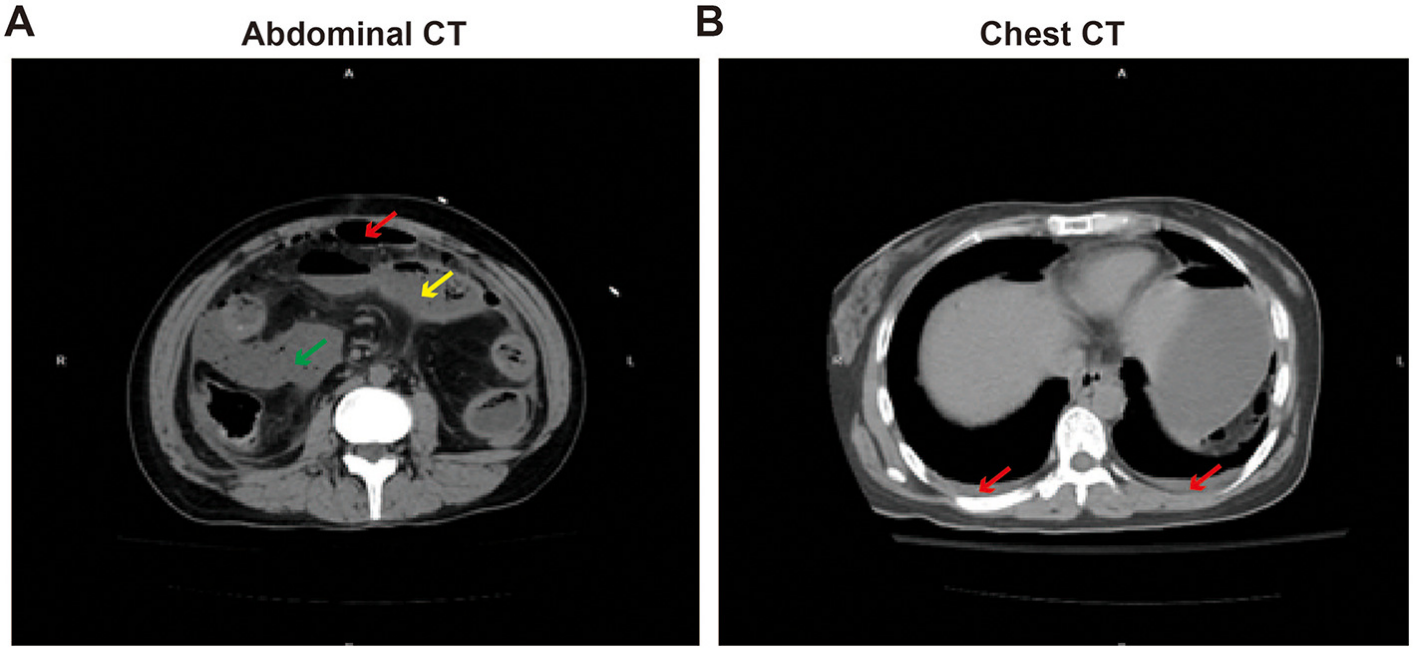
Abdominal and chest CT imaging diagnosis. (A) Abdominal CT, The red arrow mark free gas in the abdominal cavity and perforation of the gastrointestinal tract; yellow arrow indicates mild intestinal distension and fluid presence; the green arrow indicates the presence of peritoneal effusion. (B) Chest CT, the red arrow indicates a small amount of pleural effusion.
